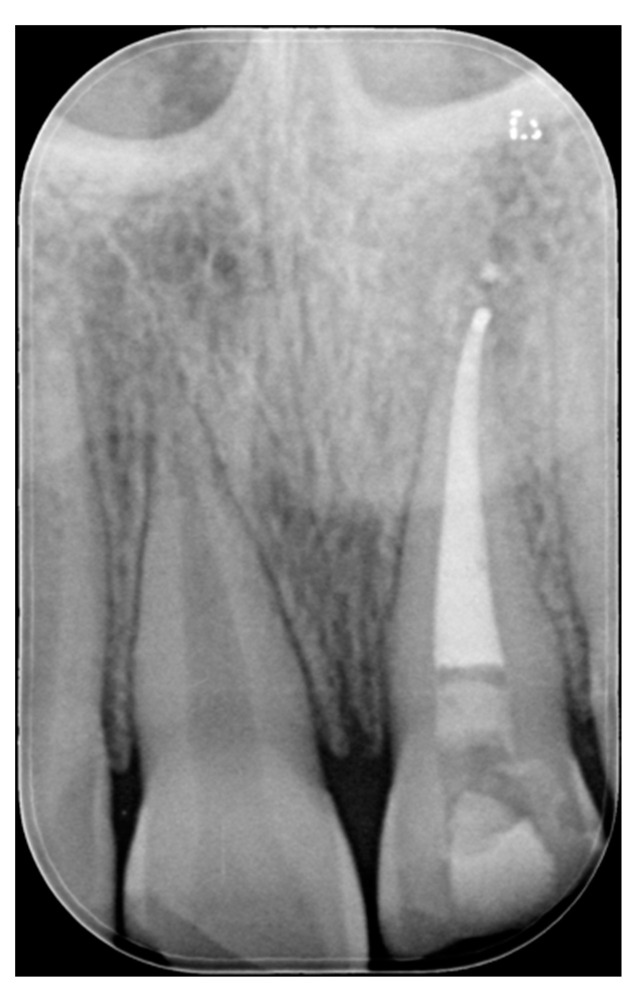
X-ray after endodontic treatment. Reprinted from Restauri diretti nei settori anteriori, G. Paolone, S. Scolavino, © 2021, with permission from Quintessence Publishing Italy.
